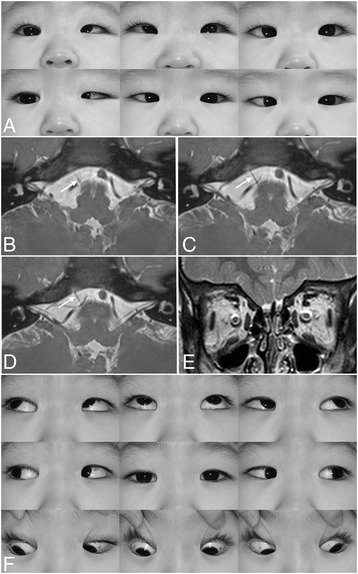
Duane retraction syndrome type 1 in the left. a Ocular versions demonstrating limited abduction, fissure narrowing on attempted adduction and a small upshoot in the left eye. b-d Three consecutive thin-section T2-weighted images show the normal right abducens nerve (arrows) as a dark structure emerging from the pontomedullary junction (b), coursing superiorly and obliquely (c), and finally entering the Dorello’s canal (d). The left abducens nerve is not identified. e Coronal T2-weighted image of the orbit shows extraocular muscles in normal size. f Ocular versions demonstrating full abduction and a small upshoot in the left eye
